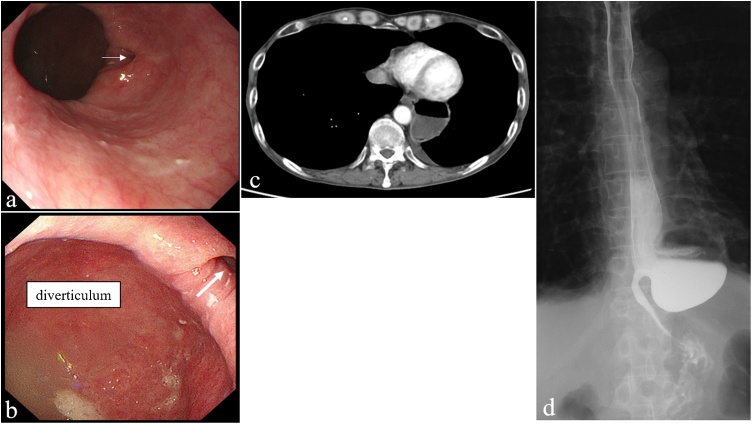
Preoperative imaging findings. a, b: Upper gastrointestinal endoscopy findings. There was a giant esophageal diverticulum at the lower thoracic esophagus and stenosis in the anal side of the diverticulum (arrow). c: CT findings. There was a 54 mm esophageal diverticulum at the lower thoracic esophagus and fluid collection within the diverticulum. d: Upper gastrointestinal contrast study findings. The entrance of the diverticulum was located on the left side of the lower esophagus, and pooling of gastrografin in the diverticulum and delayed passage into the stomach were observed.
